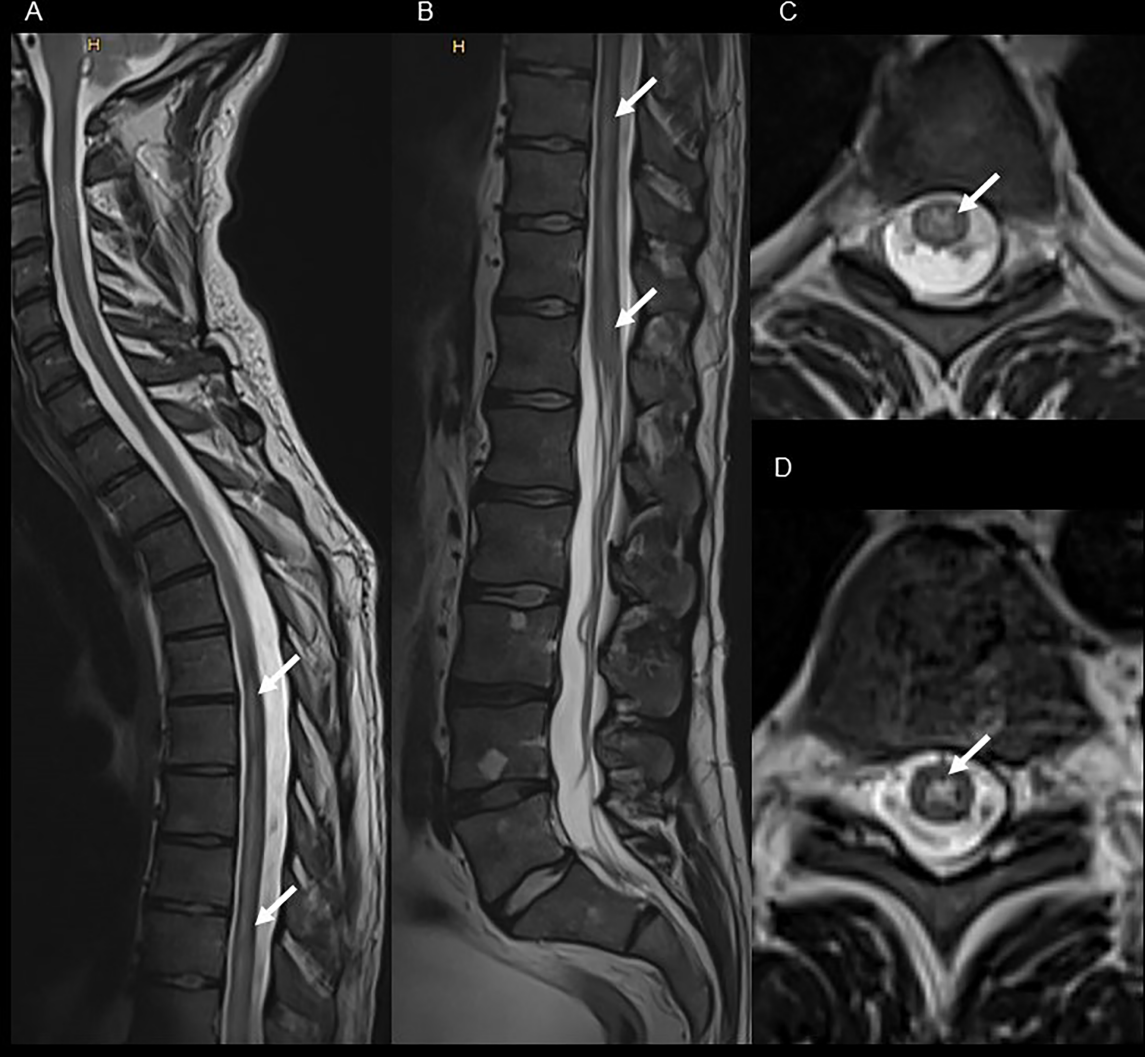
Spinal MRI of the index patient presenting with MOG-IgG associated LETM following VZV infection. T2 weighted cervical, thoracic [panel (A), sagittal view] and lumbar [panel (B), sagittal view] spinal cord MRI shows extensive hyperintense lesion from the level T1 to the conus medullaris [panel (C), axial view of T4; and panel (D), axial view of T9] with only a very faint contrast enhancement (not shown).
